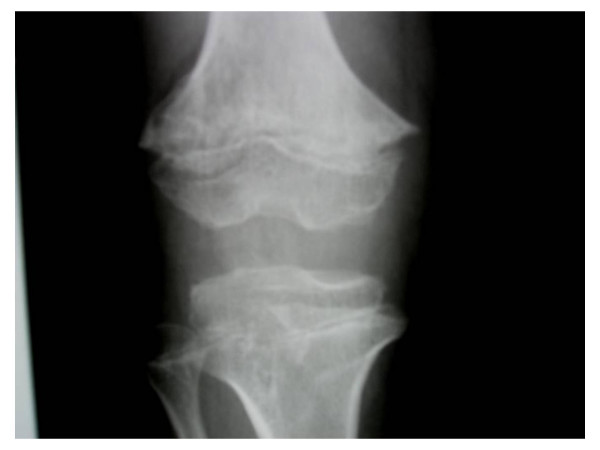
Anteroposterior radiograph of the knee showing multiple small enchondroma-like metaphyseal dysplasias. The distal femoral and the proximal tibial bones show metaphyseal cupping with multiple enchondromatous lesions and an abnormal metaphyseal trabecular pattern associated with small round rings and arcs and dense foci is as intended here within the metaphysis.
